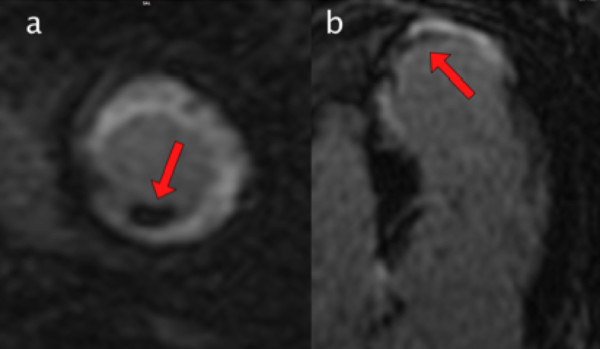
Gadolinium enhanced CMR viability image.a. Left ventricular short axis mid-papillary orientation b. Apical 4 chamber orientation. Mural thrombus with low signal intensity (arrow) adjacent to blood pool and myocardial scar.
